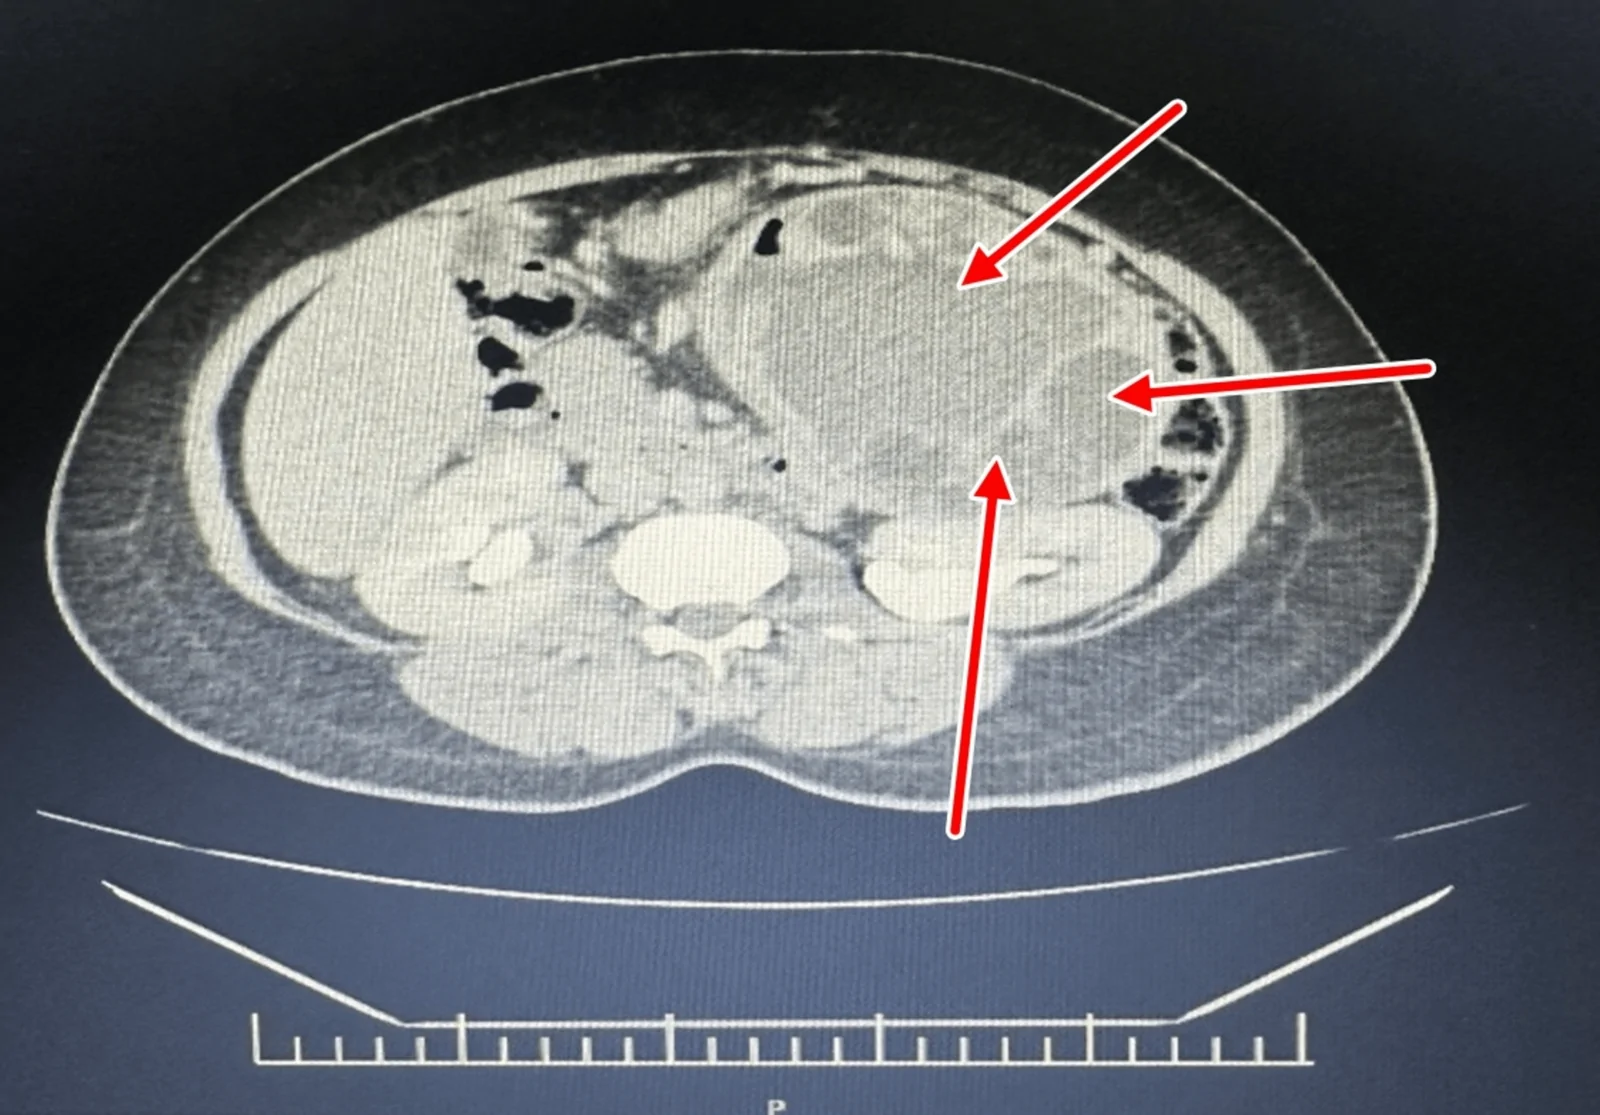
Arterial-phase CECT image of the abdomen demonstrating multiple enhancing internal septa within the cystic lesion (red arrows indicate the enhancing internal septa) CECT: Contrast-enhanced CT
